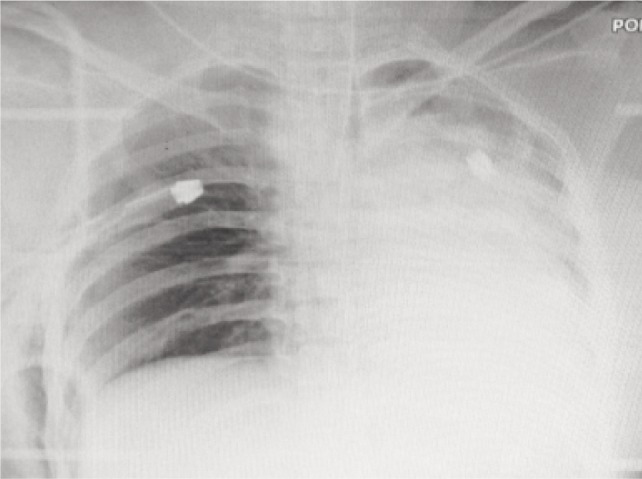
Chest radiograph with ribs fractures and pneumothorax.
